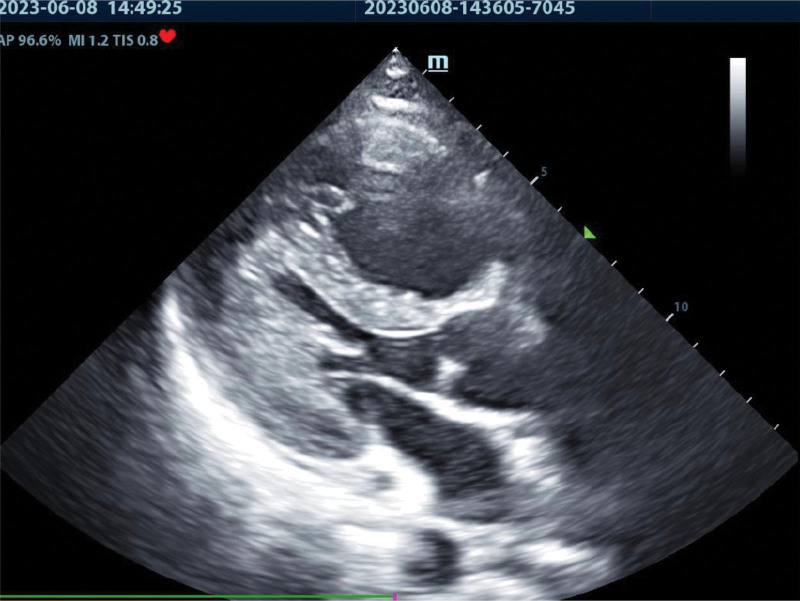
Cardiac ultrasound: the right heart was enlarged, the left heart became smaller, and the cardiac activity was weak, which was consistent with the ultrasonic changes of pulmonary embolism.
